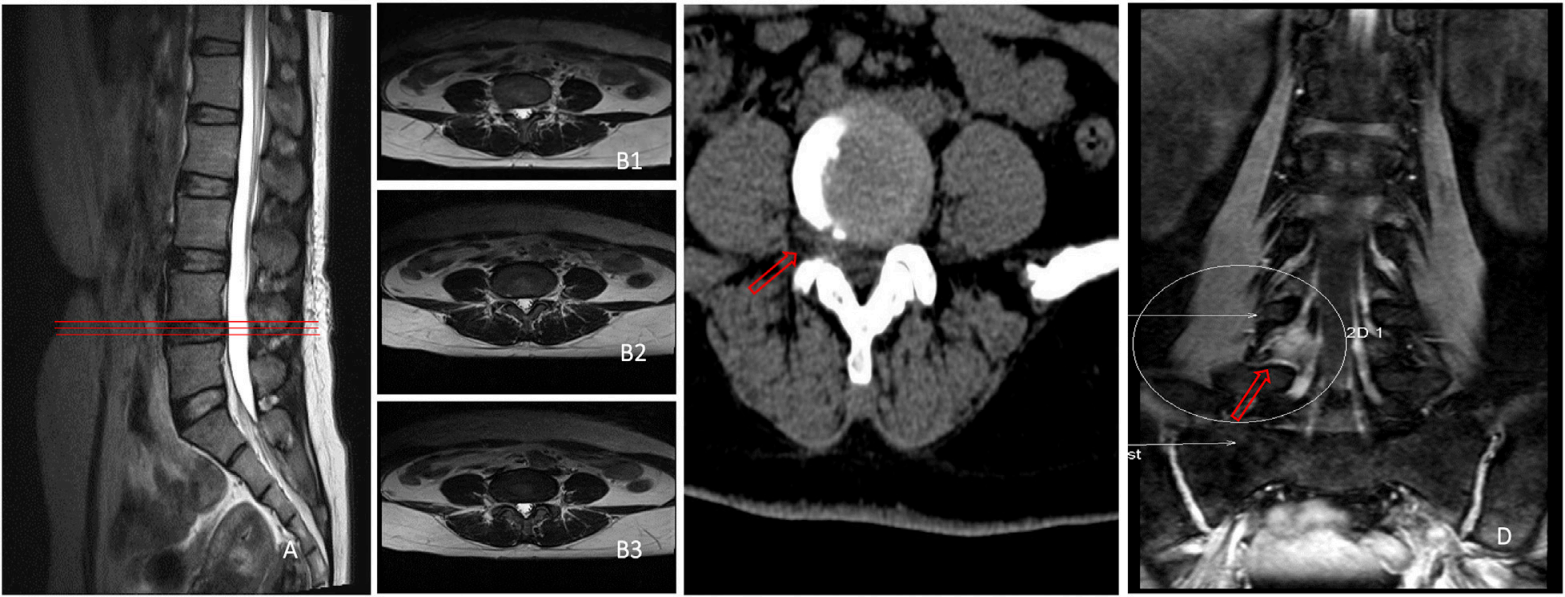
No suspicious disc herniation was found in L4/L5 segment in both sagittal (A) and cross-sectional MRI (B1–B3), only one suspicious herniated nucleus pulposus existed on the extreme lateral side of L4/5 segment in cross-sectional CT (C), and a huge nucleus pulposus could be seen to compress the right L4 nerve root under the armpit in 3D-DESS (D).
